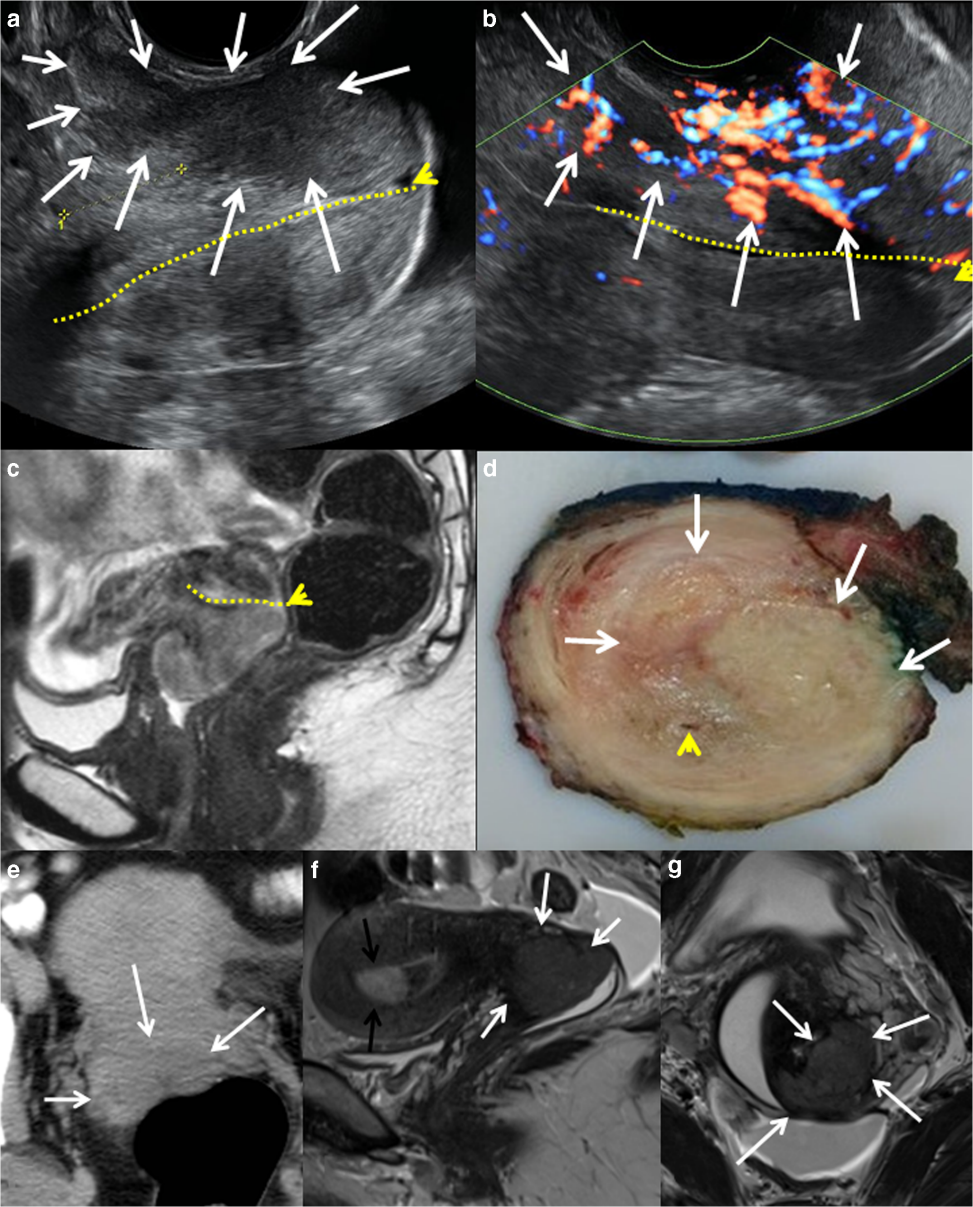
Bulky cervical cancer depicted by grayscale TRS (a), color Doppler TRS (b), and sagittal T2-weigthed MRI (c) in a 26-year-old patient with squamous cell carcinoma, clinical FIGO stage 1B2. The cervical cancer lesion (white arrows) appears hypoechoic on TRS (a) and with high perfusion on color Doppler (b). The same tumor is hyperintense on T2-weighted MRI (c). After surgical removal macroscopic transversal section (d) shows tumor infiltrating the anterior lip of the cervix (white arrows), while the endocervical canal (yellow line) and posterior labium is intact (yellow tip showing external cervical os). Axial contrast-enhanced CT (e) depicting a slightly hypodense cervical lesion (arrows) relative to the surrounding stroma in a 54-year-old patient with squamous cell carcinoma, clinical FIGO stage 1B1. Sagittal (f) and paraaxial (g) T2-weighted MRI depicting a hyperintense cervical lesion (arrows) in a 41-year-old woman with squamous cell carcinoma, clinical FIGO stage 1B1 cervical cancer (same patient as in Fig. 2 and Fig. 3a, b). The incidentally detected hyperintense lesion in the uterine cavity (f, black arrow) was diagnosed as a benign endometrial polyp
